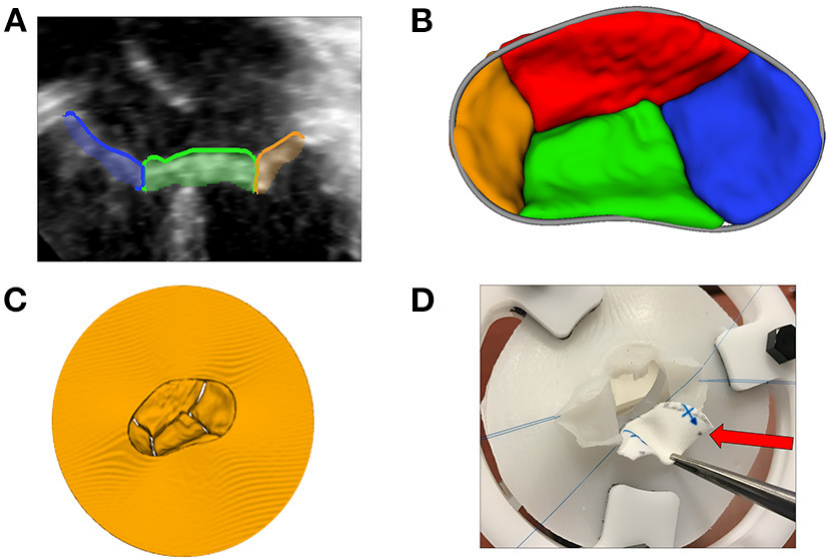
3D printing and novel visualization technologies. (A) A 2D transthoracic apical view of a CAVC valve (from image left to right: right mural leaflet in blue, inferior bridging leaflet in green, and left mural leaflet in orange) segmented using the Valve Segmentation module in SlicerHeart; (B) An 3D atrial view of a segmented CAVC valve with the annular curve visible in gray; (C) A virtual model of the segmented CAVC valve with a skirt used as a template for 3D printing; (D) A surgeon practices a patch repair on a AVC valve created from a 3D echocardiogram with the dividing ventricular septal defect patch indicated by the red arrow. CAVC, complete common atrioventricular canal.
